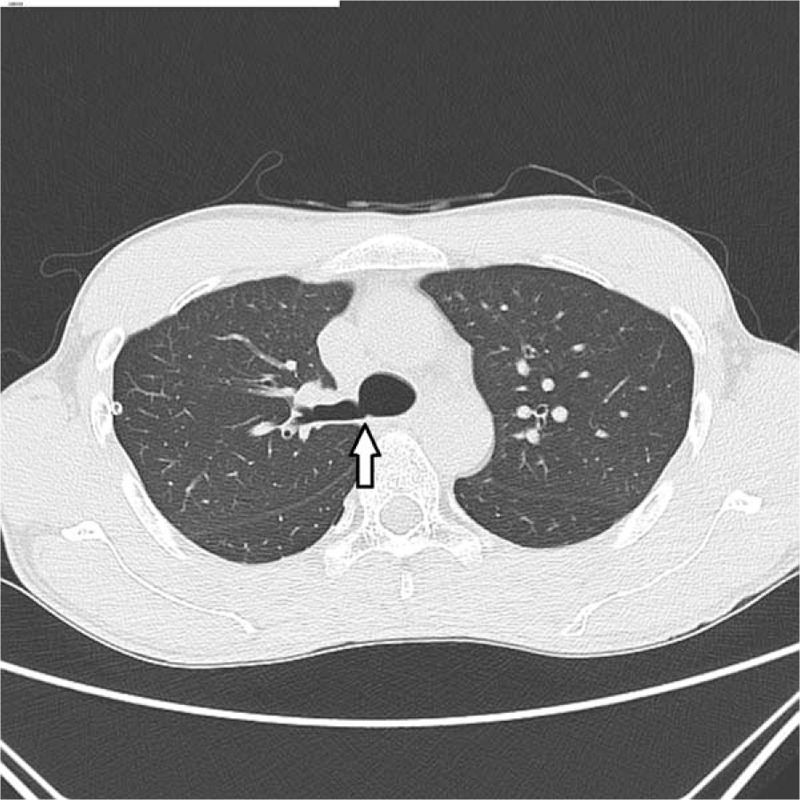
Preoperative axial chest computed tomography image shows acute angulation of the branching portion of the tracheal bronchus, mimicking the carina (arrow).
